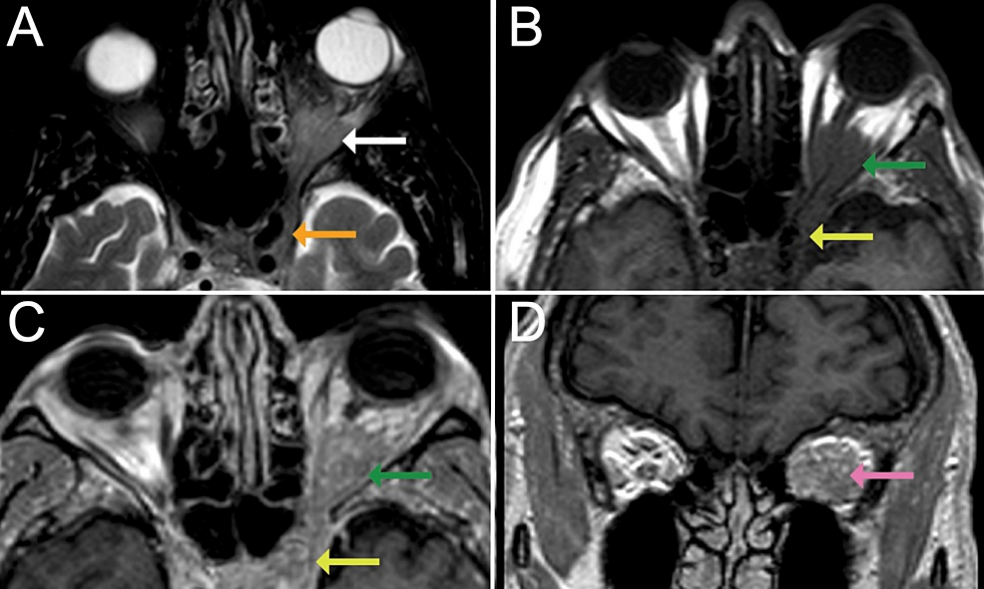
Brain MRI images. T2 weighted Brain MRI axial sequence demonstrating a T2 hypointense lesion (white arrow) centered in the mid- to posterior intraconal compartment of the left orbit involving the intraocular muscles near the region of the orbital apex. (A) The lesion is seen compressing the optic nerve sheath displacing it superomedially. Similar signal intensity is observed within the left cavernous sinus consistent with sinus invasion (orange arrow). Axial (B) pre- and (C) post-contrast T1 weighted sequences demonstrating homogeneous enhancement of the orbital lesion (green arrow) with associated proptosis and extension to the cavernous sinus (yellow arrow). (D) Coronal post-contrast T1 weighted MR sequence demonstrating homogeneous enhancement of the orbital lesion (pink arrow).
